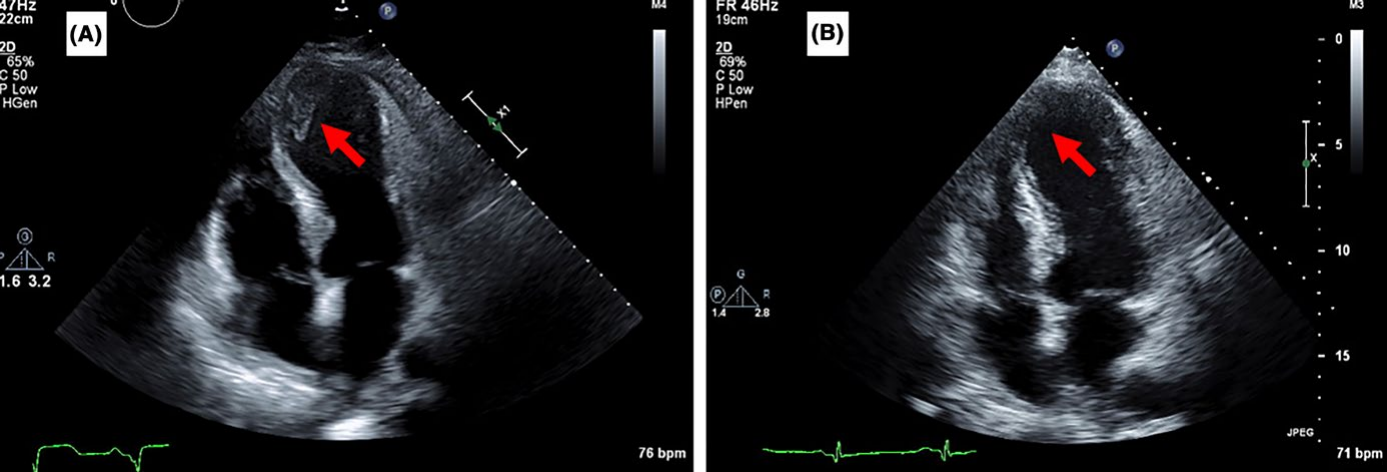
A, Apical four chamber view showing the LV thrombus of case one. B, Resolution of the thrombus after three months of anticoagulation with Rivaroxaban
